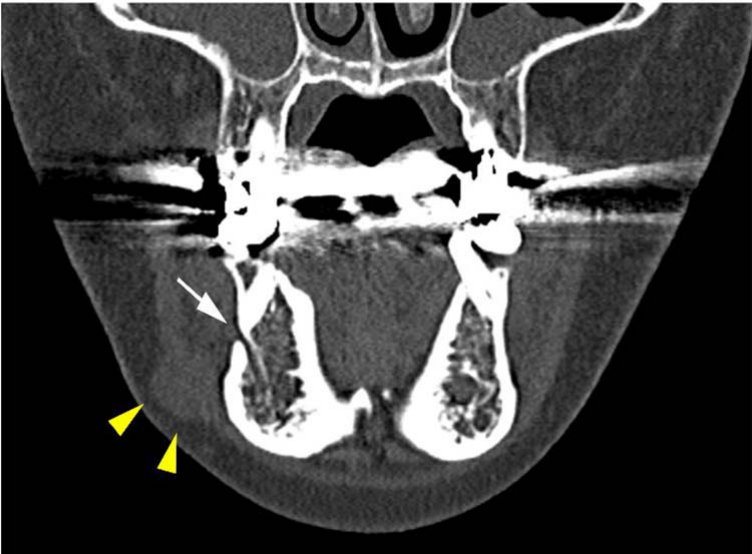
CT findings of a coronal section; yellow arrowheads indicate the schwannoma; the white arrow indicates the mental foramen.
